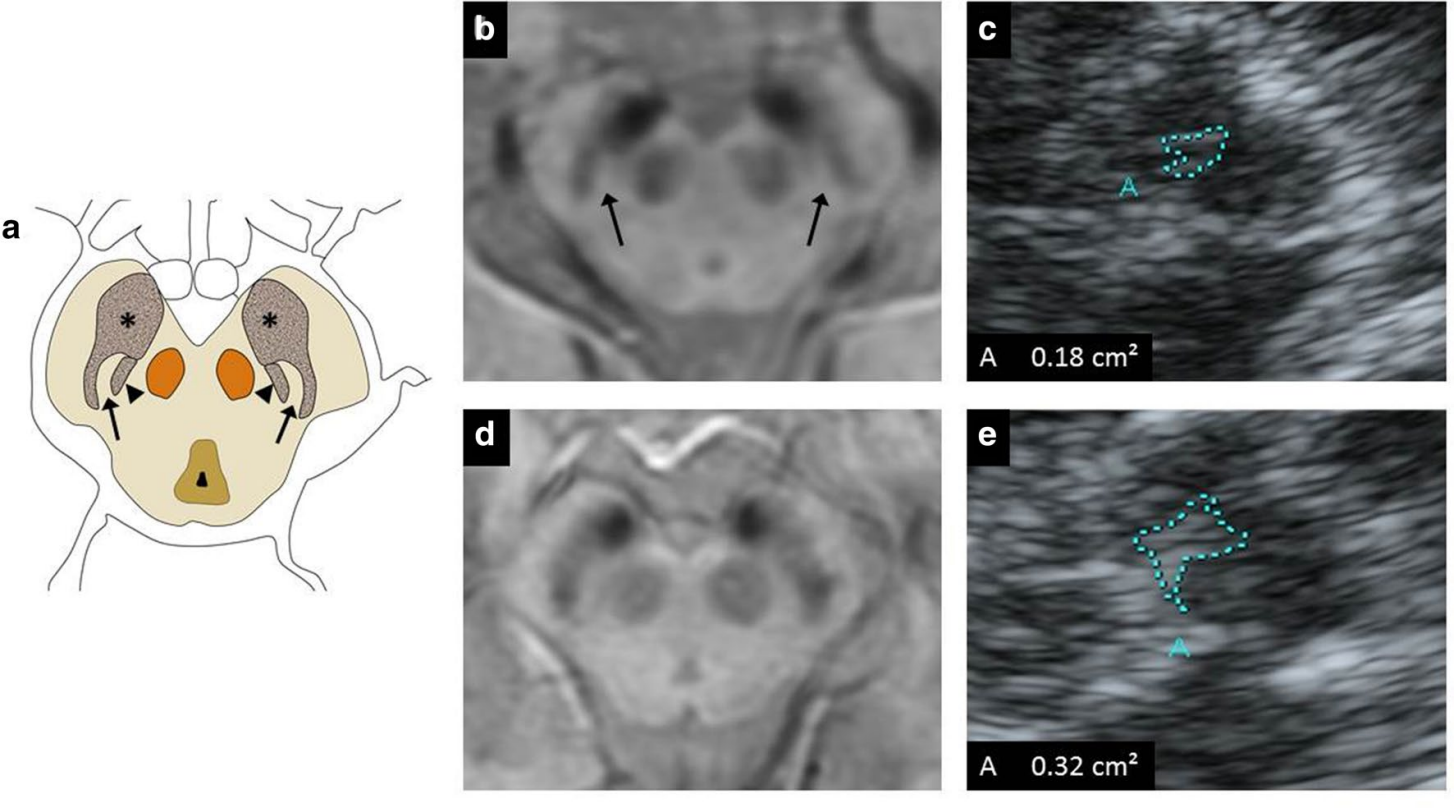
Anatomic representation of mesencephalon including nigrosome 1 and its surrounding structures (a, *asterisk* = substantia nigra; *arrowhead* = medial lemniscus; *arrow* = nigrosome 1; *circle* = red nucleus). Nigrosome 1 present on susceptibility weight images of MRI (b, *arrow*) and area of the SNh (c). Nigrosome 1 absent on susceptibility weight images of MRI (d) and larger area of SNh (e). ALS: amyotrophic lateral sclerosis; SNh: substantia nigra hyperechogenicity
